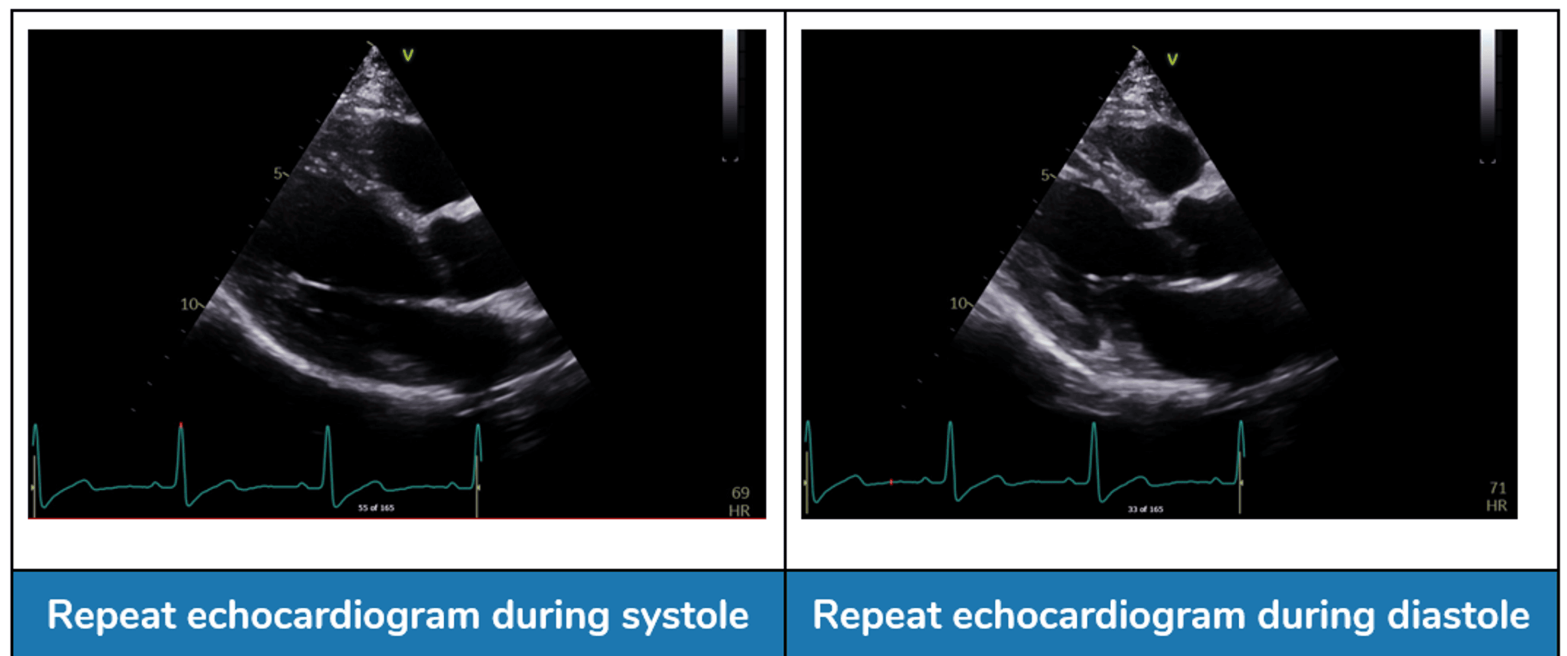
Repeat echocardiogram (five months after initial presentation). Repeat echocardiogram at five months post discharge showed significant recovery of left ventricular (LV) systolic function (biplane left ventricle ejection fraction (LVEF): 45–50%, triplane LVEF: 50%), with mild residual grade 1 diastolic dysfunction (E/A ratio: 0.8, deceleration time: 220 ms) and a persistent false tendon in the apical region, confirming the reversible nature of takotsubo cardiomyopathy.
